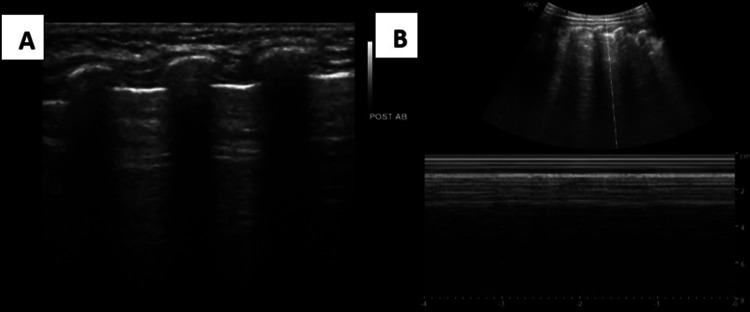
A) B-mode ultrasound in the left posterior upper region shows normal pleural line and A-lines; B) M-mode ultrasound showing “Sand on the Beach” appearance. B mode: Brightness mode; M mode: Motion mode
